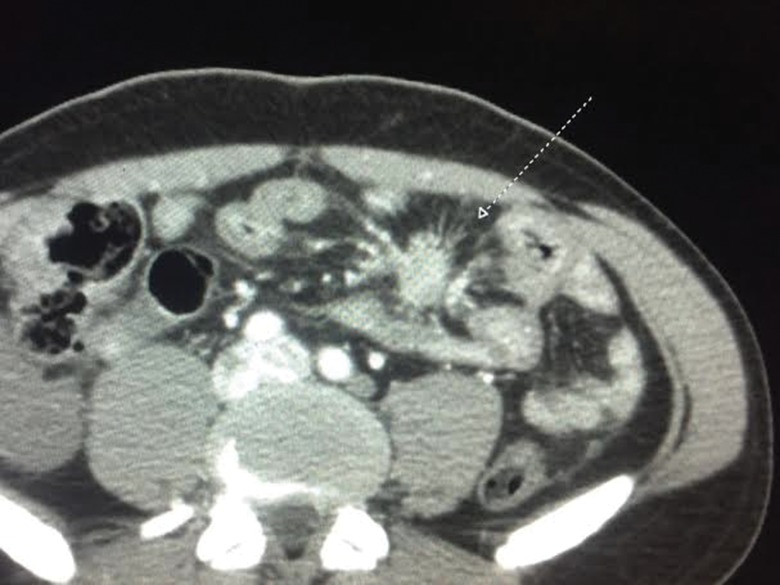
CT showing a characteristic image of mesenteric fibrosis.
